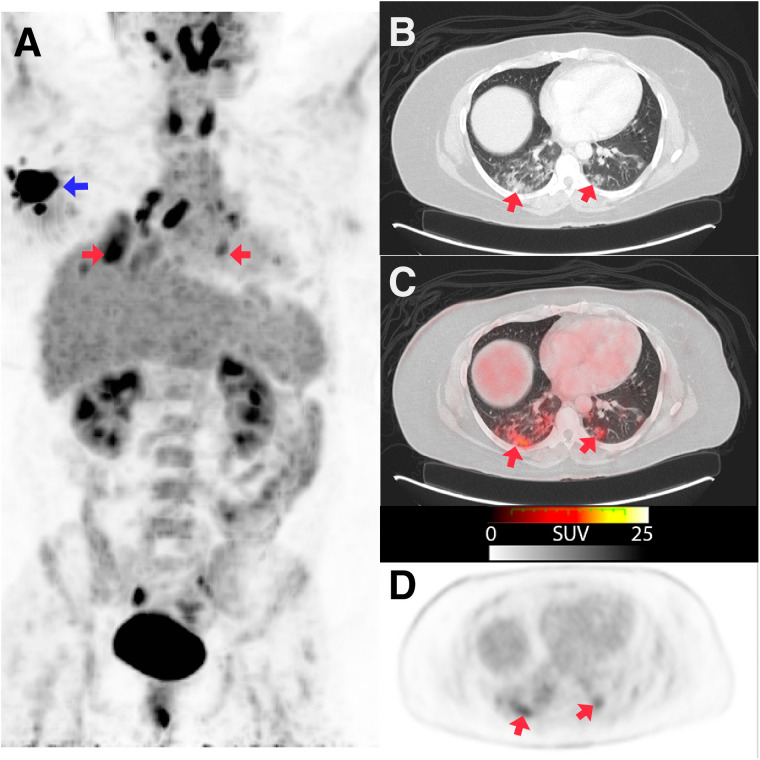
Staging PET/CT of 43-y-old woman with breast cancer (A, blue arrow). Maximum-intensity projection (A) and axial sections with lung window (B) and fusion (C) images show bilateral ground-glass pulmonary infiltrates (red arrows), some of them with pseudonodular morphology, located in both lower lobes and left middle lobe, with diffuse or peripheral distribution (SUV_max_, 7.8). Early rRT-PCR obtained in emergency department was negative for COVID-19, and second test was not available.
